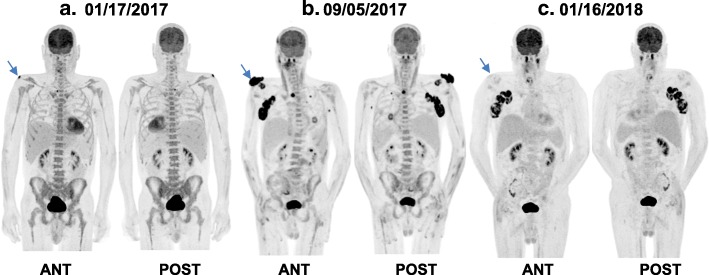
Anterior and posterior volume-rendered maximum activity-reprojection FDG-PET images showing (a) FDG uptake in the right shoulder (arrow) and a paratracheal lymph node. b markedly hypermetabolic lesion in the right shoulder with SUV_max_ of 26.7 (arrow), markedly hypermetabolic right axillary and right paratracheal lymphadenopathy with SUV_max_ of 27.3 with interval development of hypermetabolic focal cutaneous lesions, left axillary lymph node and pulmonary lesions. c near complete response of the right shoulder hypermetabolic lesion (arrow), complete resolution of paratracheal lymph node, left axillary lymph node, pulmonary and cutaneous lesions, with persistent FDG uptake of the right axillary lymph nodes
